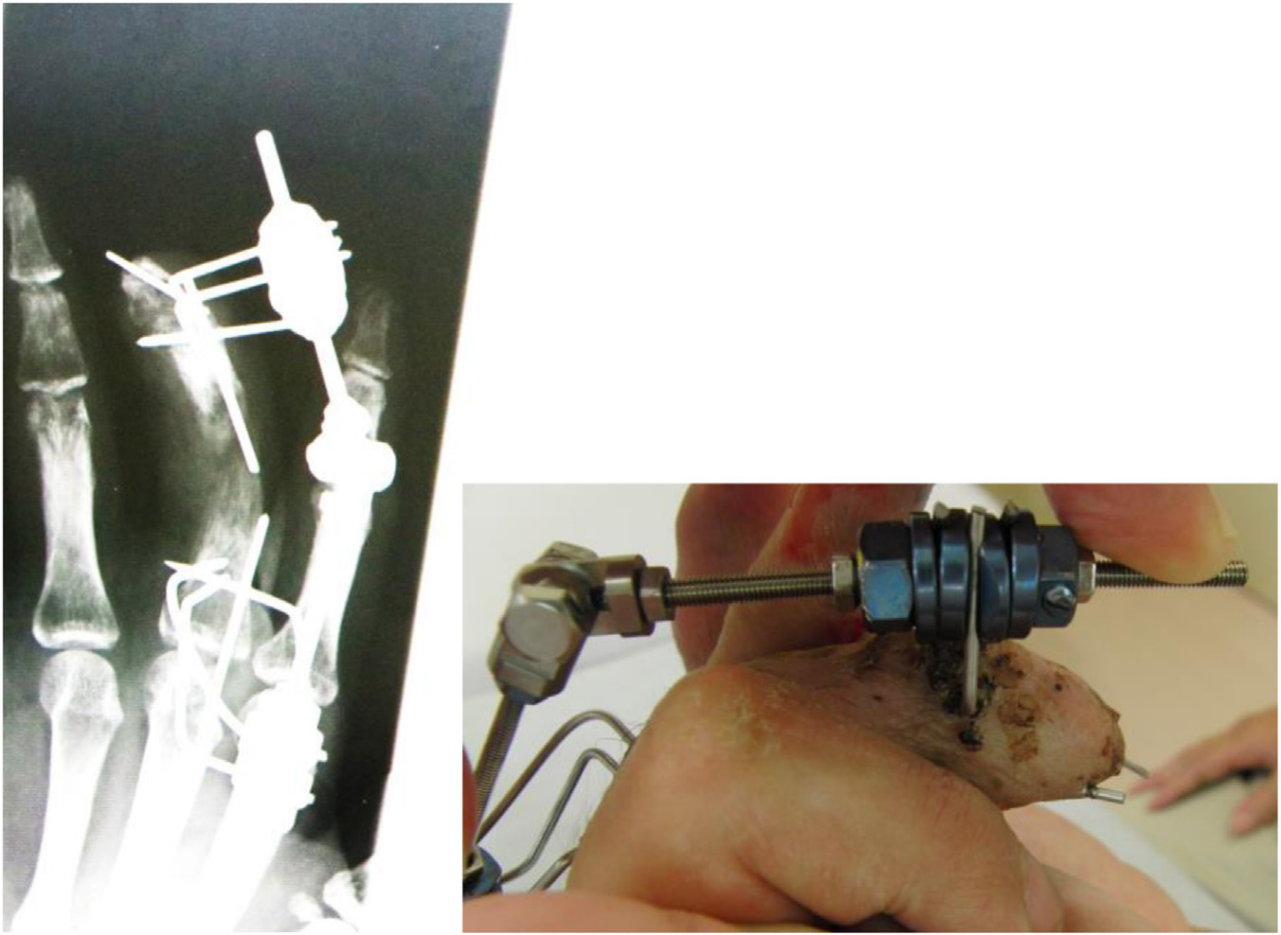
(Left) X-ray shows two wires below the hinge. (Right) Hinge is shown between two M3 units.
